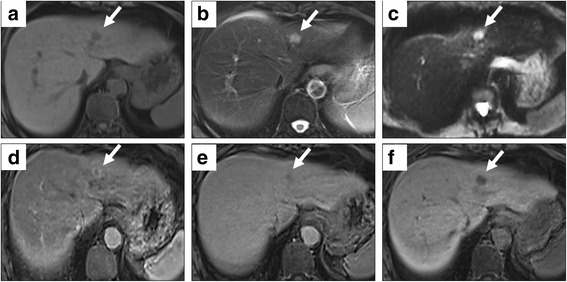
Findings of magnetic resonance imaging (MRI). The tumor shows low intensity in T1-weighted image (a) and shows high intensity in T2-weighted image (b). The tumor exhibits marked high intensity on the diffusion-weighted image (c). Dynamic MRI shows peripheral enhancement of the tumor in the early phase (d) and low intensity relative to the normal liver in the late phase (e). In the hepatobiliary phase, the tumor exhibits defects in enhancement (f)
